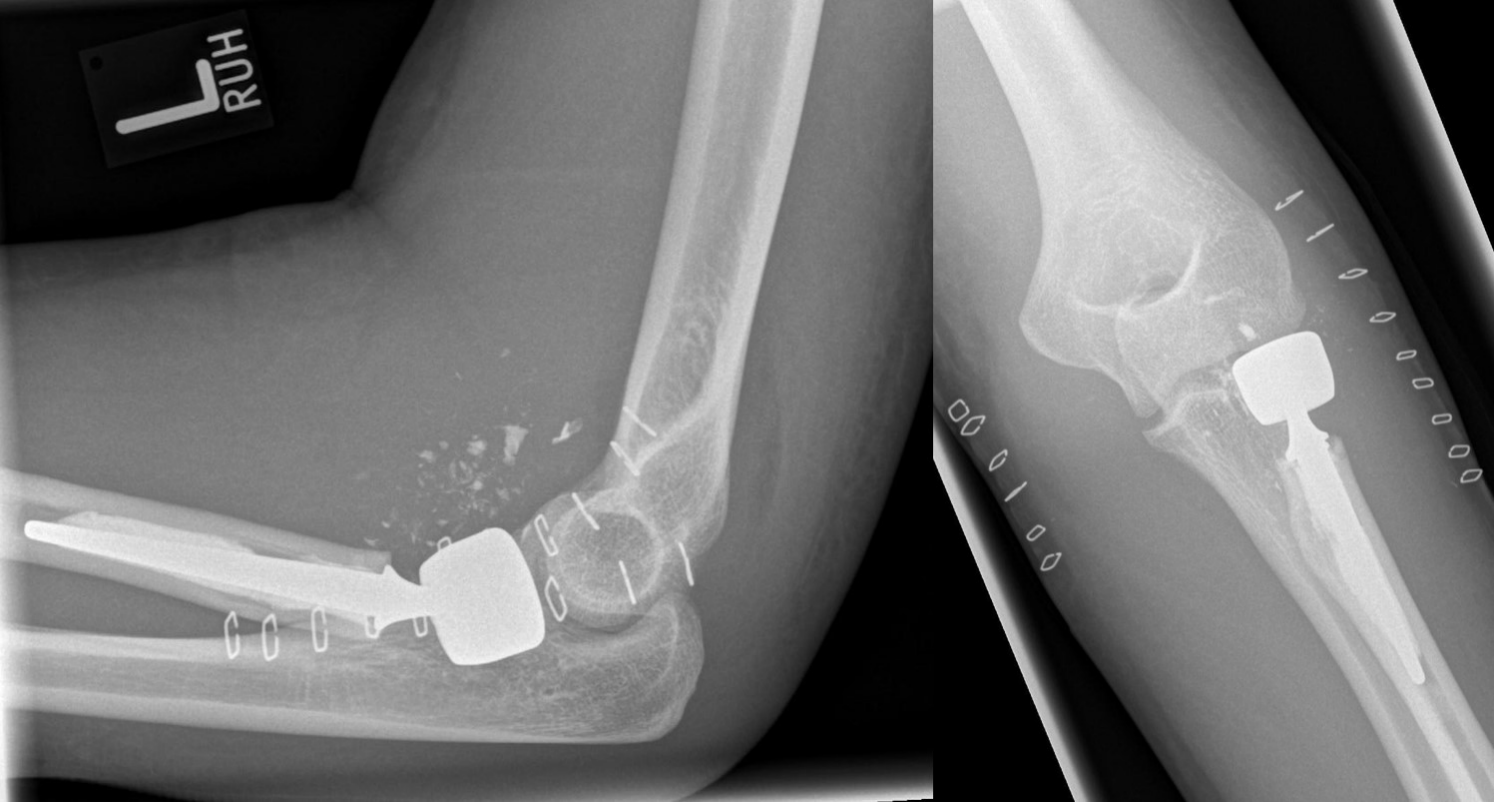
Postoperative radiographs after implantation of a cemented bipolar radial head prosthesis
